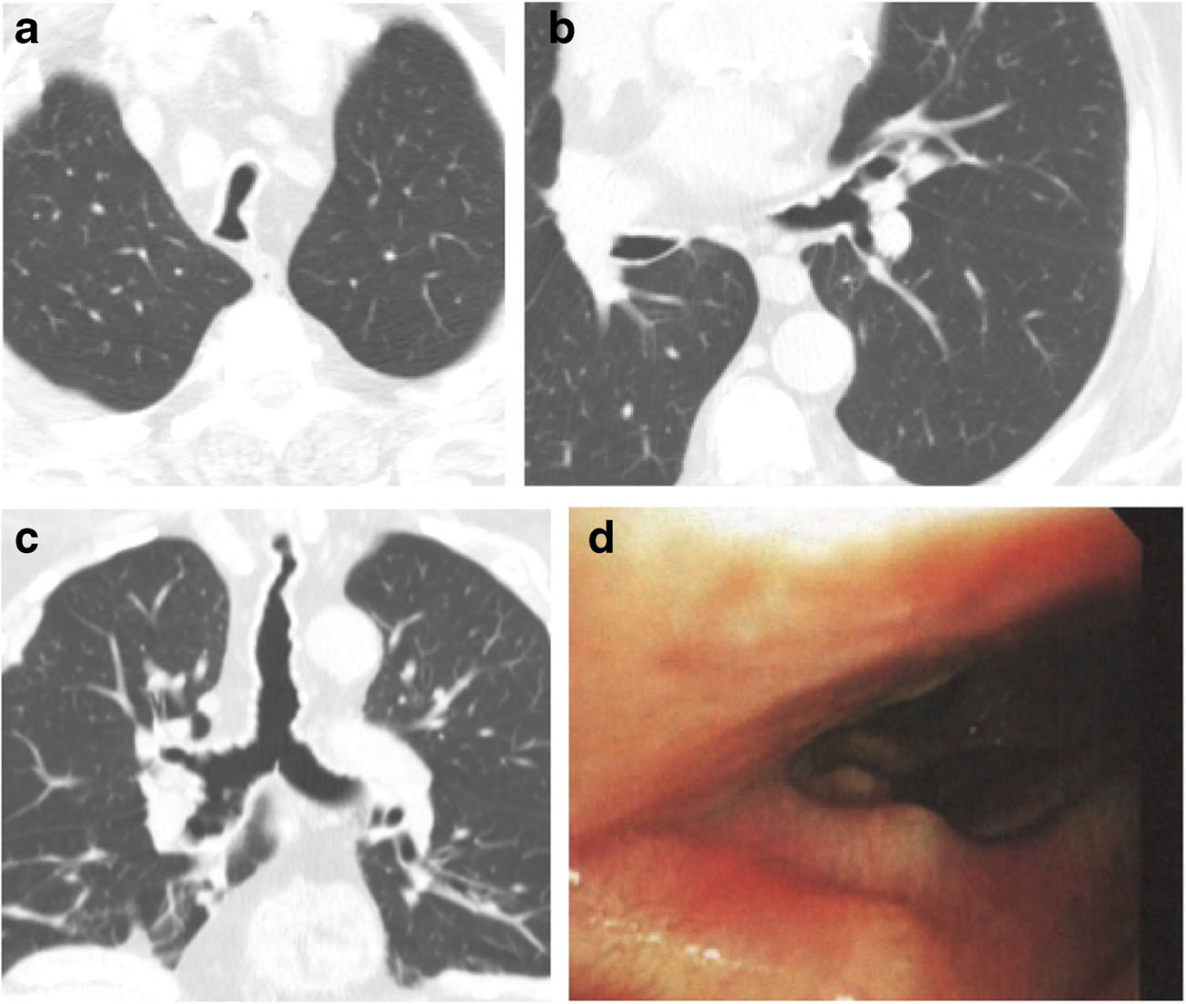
Eighty-two-year-old man with axial a, b and coronal c CT imaging demonstrating narrowed tracheal caliber and irregularity of the walls of the trachea, extending into the bilateral mainstem bronchus compatible with tracheobronchopathia osteochondroplastica. d Video still at the time of bronchoscopy demonstrating luminal narrowing of the trachea
